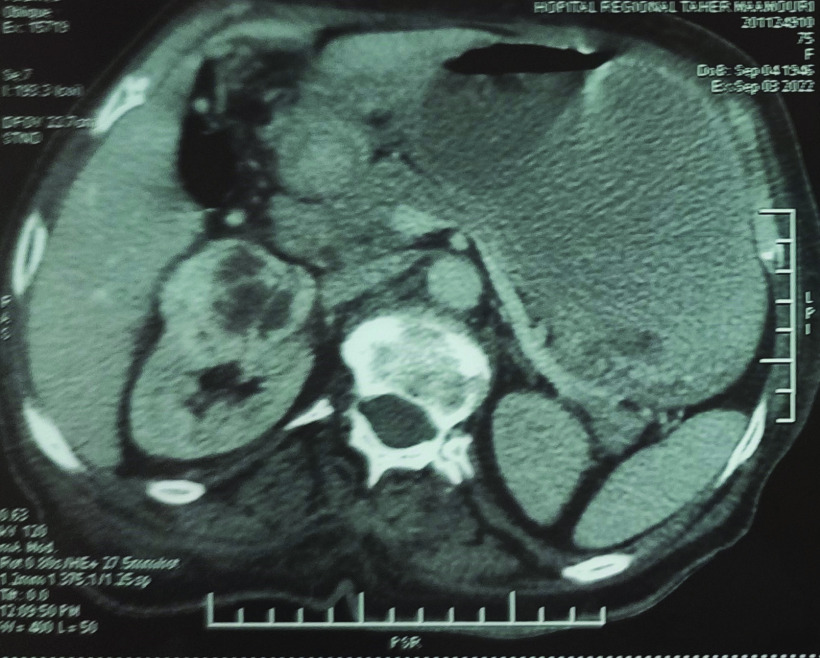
A computed tomography image showing an exophytic hypervascular mass of the inferior pole of the left kidney.
